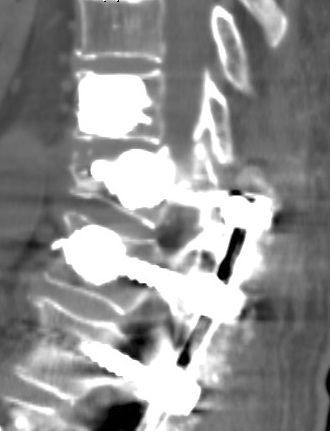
Computed tomography revealing a collapse and wedging of the T10 vertebral body and distraction pattern fracture at pedicles through the upper edge of the screw, with anterior subluxation of T9.
